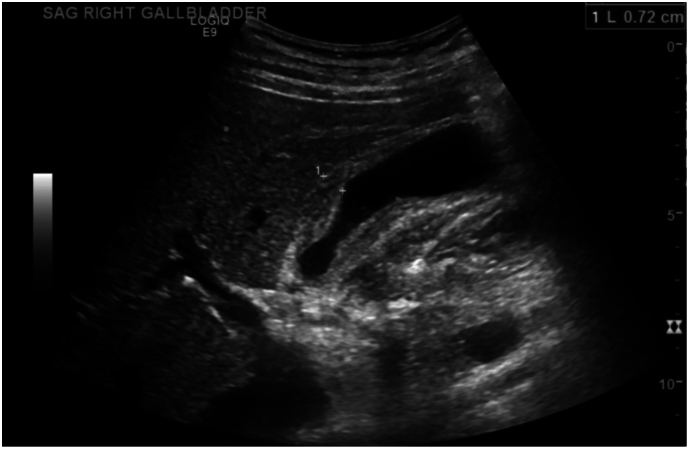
Abdominal ultrasound showing a thickened gallbladder wall with pericholecystic fluid.
